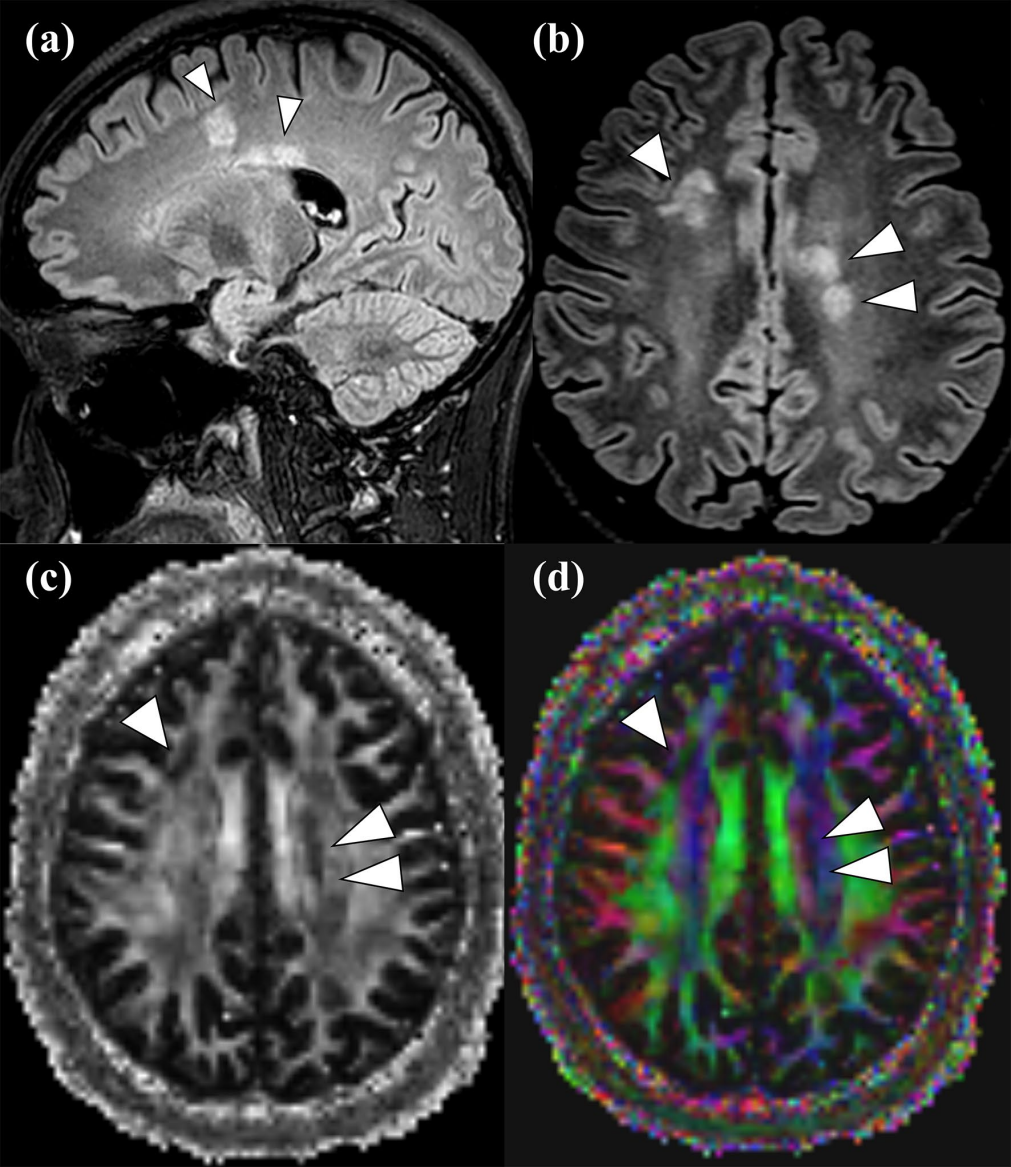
Representative patient with multiple sclerosis. A 23-year-old man presented with dysarthria and facial palsy (EDSS = 3; periependymal area of 4th ventricle: FA = 0.764, AD = 0.000643; periependymal area of 3rd ventricle: FA = 0.563, AD = 0.00134; periependymal area of left lateral ventricle: FA = 0.782, AD = 0.0004; and periependymal area of right lateral ventricle: FA = 0.765, AD = 0.00034). Note the multifocal periependymal T2 hyperintense lesions (*arrowhead*) on 3D FLAIR image (a) sagittal view, (b) axial view, as well as decreased (*dark area*) FA values on the (c) grayscale FA map, and heterogeneous colors on the (d) FA color-coded map at corresponding lesions (*arrowhead*).
